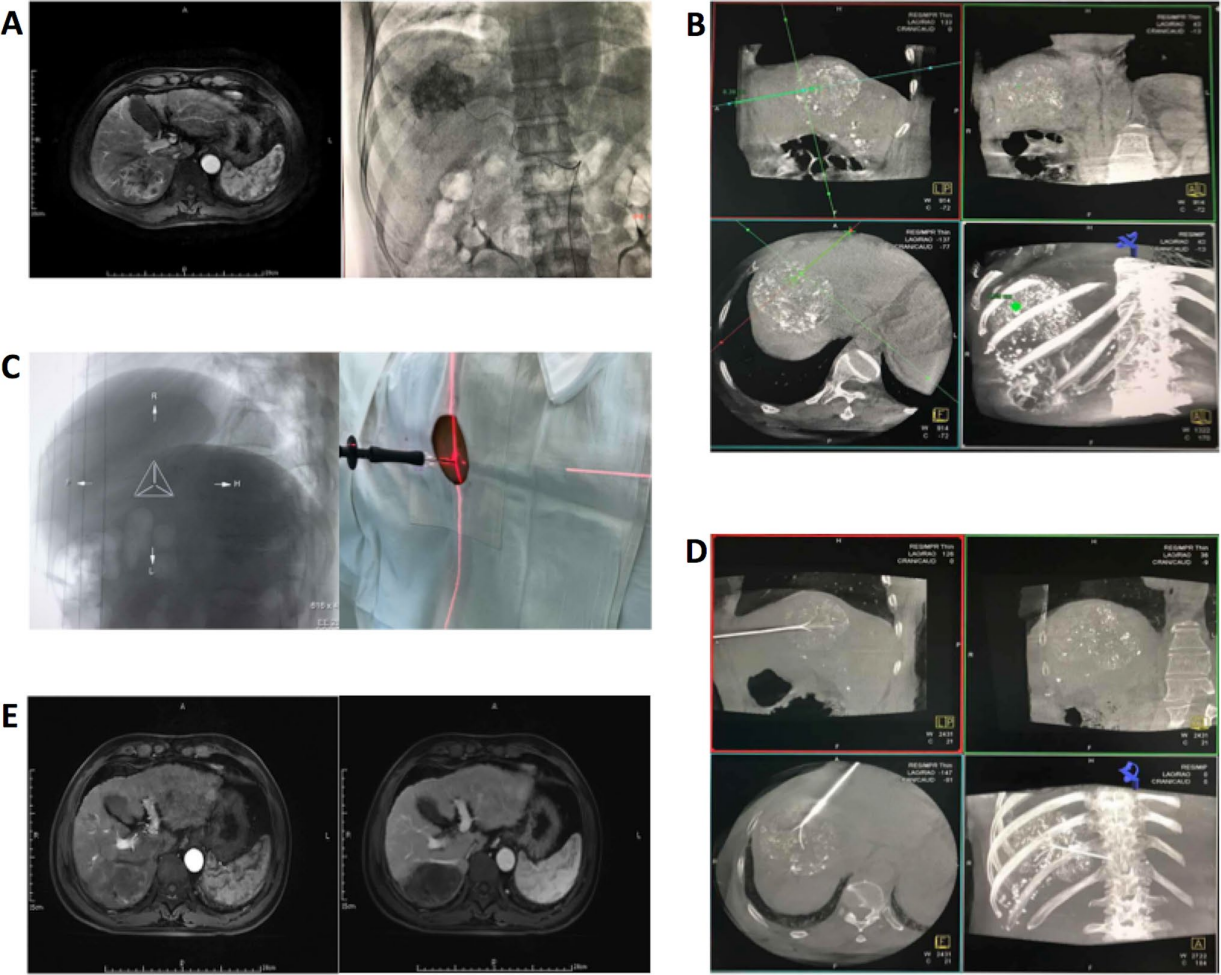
A59-year-old male. a Preoperative MRI shows a hypointense liver lesion and lipiodol embolism during TACE. b DynaCT performed immediately after TACE. The target site, surface site, and puncture routine are determined using the workstation. c Bull’s eye position; after laser localization, puncture is performed along the direction of laser. d After successful puncture, DynaCT is performed again to confirm the needle location. e MRI of the liver in the arterial phase and venous phase at 1 month after surgery
